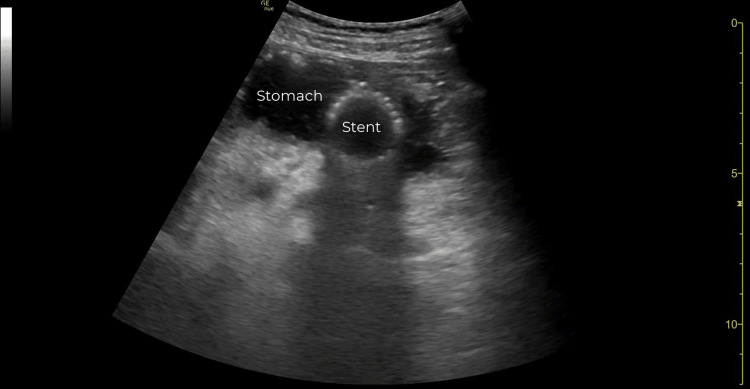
Point-of-care ultrasound of the stomach demonstrating a 2.41 cm x 2.51 cm round, echogenic, and fenestrated structure (short-axis view).
